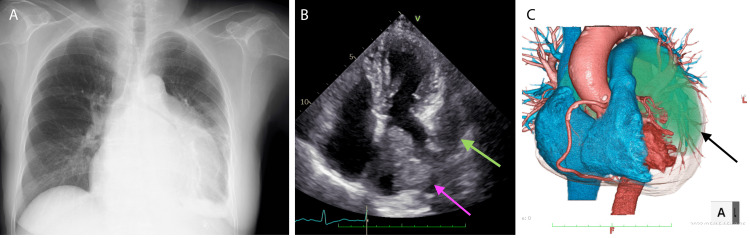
Preoperative findings. (A) Chest X-ray revealed expanding cardio-thoracic ratio. (B) Preoperative echocardiogram showed a cardiac tumor in the left atrium (pink arrow). The green arrows show the expanding tumor exceeding the left atrial wall. (C) Volume rendering computed tomography was shown. The green lesion was the cardiac tumor and was spreading to the left lateral wall (black arrow).
